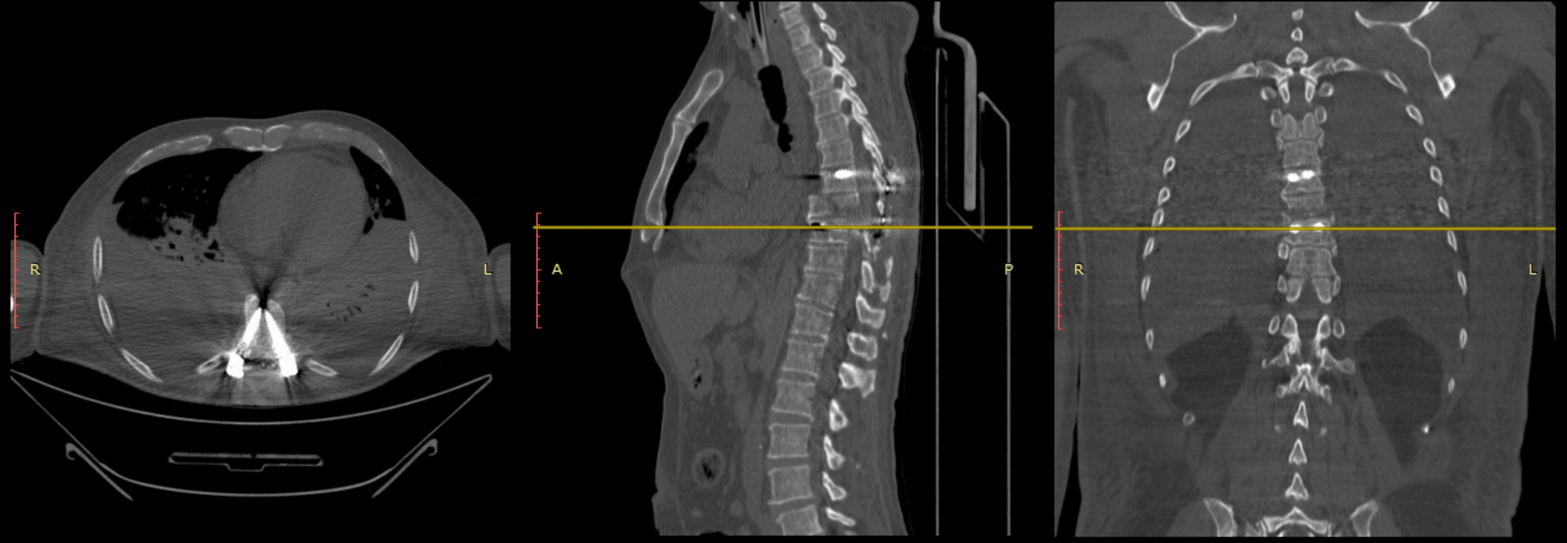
Case 3 CT scan of postoperative follow-up images showing the short-segment T8-T10 transpedicular instrumentation and the presence of bilateral hemothorax (70%).
